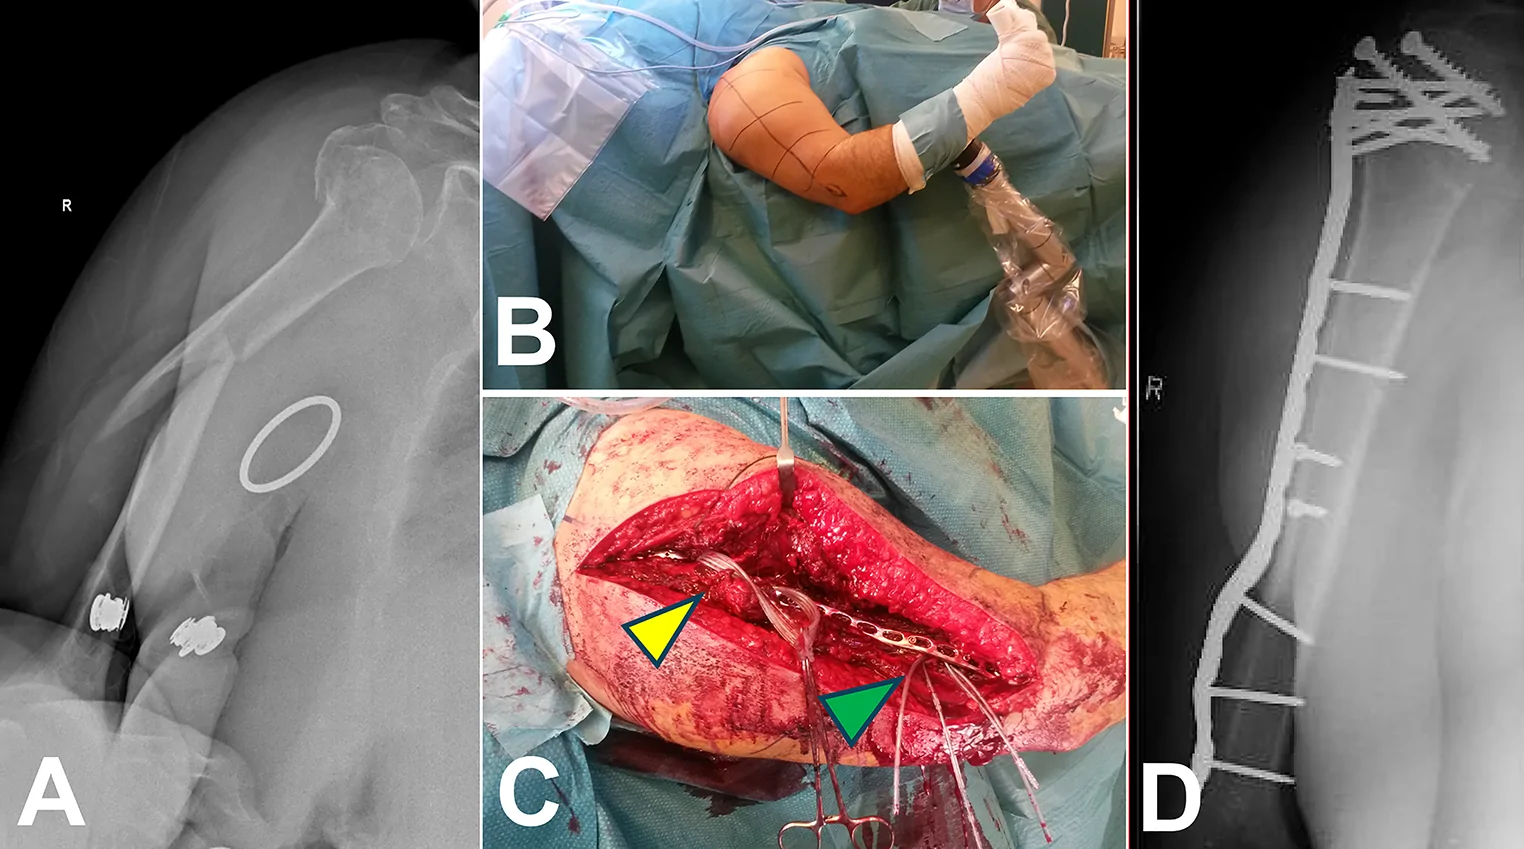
A: Preoperative x-ray of a spiral humeral shaft fracture in combination with a displaced head split fracture of the proximal humerus in a 67-year-old male. B: Intraoperative view positioning of the patient for a lateral approach. C: Intraoperative view of the lateral approach with a deltoid split and isolation of a deltoid muscle bridge containing the axillary nerve (yellow triangle) and a lateral positioned RNBP with the radial nerve and a side branch running below the wave segment of the plate (green triangle). D: Postoperative view one month after surgery
